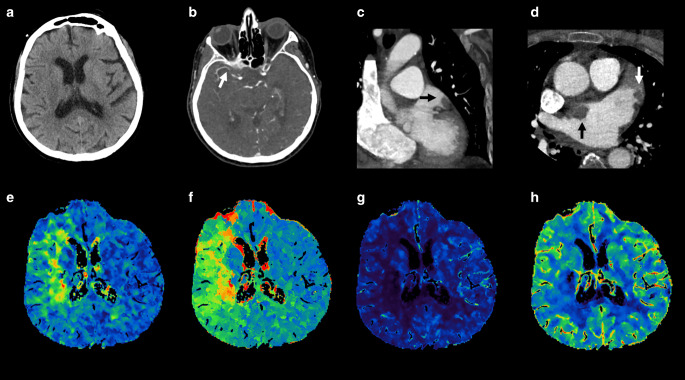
Imaging of a 73-year-old female treated endovascularly at our hospital after initial admission with suspicion of a right hemispheric stroke, occlusion of the middle cerebral artery’s first segment, and simultaneously thrombi in the left atrium and left atrial appendage. a Non-enhanced brain computed tomography (*CT*) without any stroke signs, b CT angiography showing thrombosis of the right middle cerebral artery’s first segment (*white arrow*), c coronal, and d axial heart scans show a thrombus in the left atrium (*black arrow*) and left atrial appendage (*white arrow*). The perfusion parameters show e increased mean transit time and f time to peak with no significant alteration of g the cerebral blood flow and h the cerebral blood volume in the right middle cerebral artery’s perfusion territory
